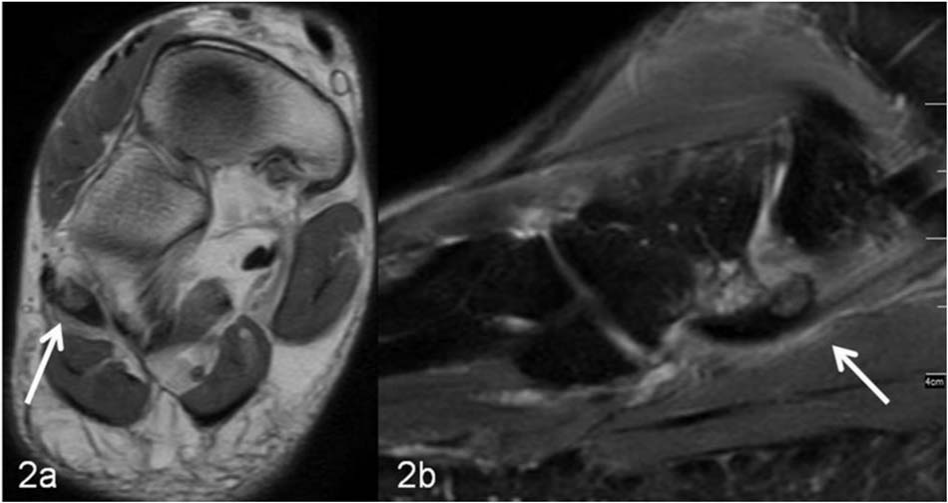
(a, b) MRI imaging, (a) axial T1 weighted slice, the os peroneum is centrally located in the long peroneal tendon (marked with an arrow). In (b) sagittal T2 weighted fat-suppressed slice, around the os peroneum (marked with an arrow) and along the tendon there is a marked signal enhancement, corresponding to inflammatory fluid accumulation (tenosynovitis).
